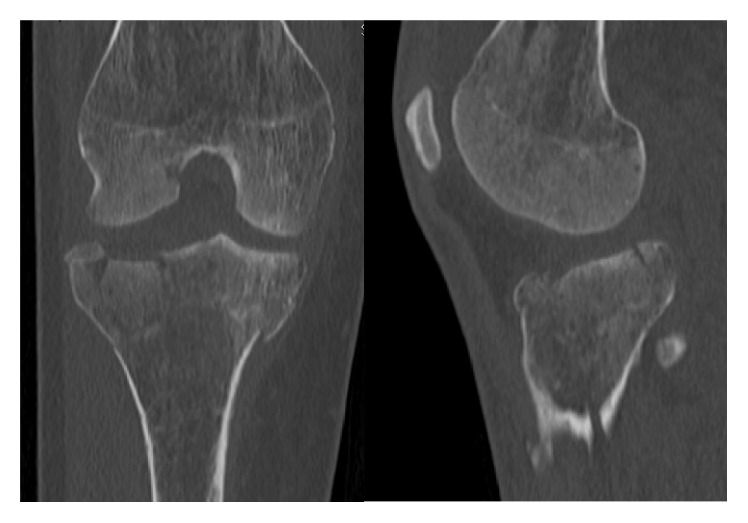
Initial CT-scan of the right knee.
